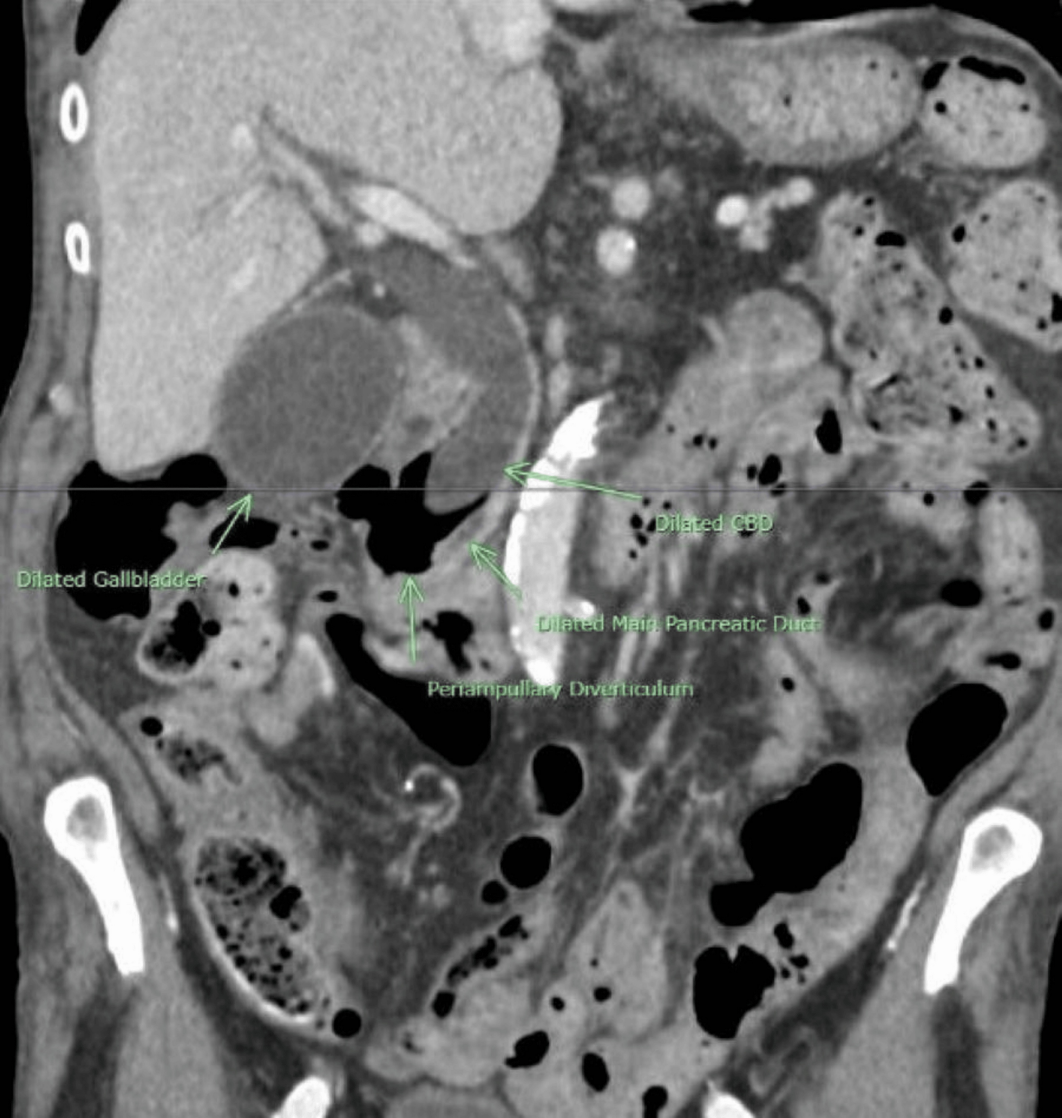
Coronal contrast-enhanced CT of the abdomen showing a periampullary diverticulum compressing the distal CBD with biliary and pancreatic ductal dilation.
